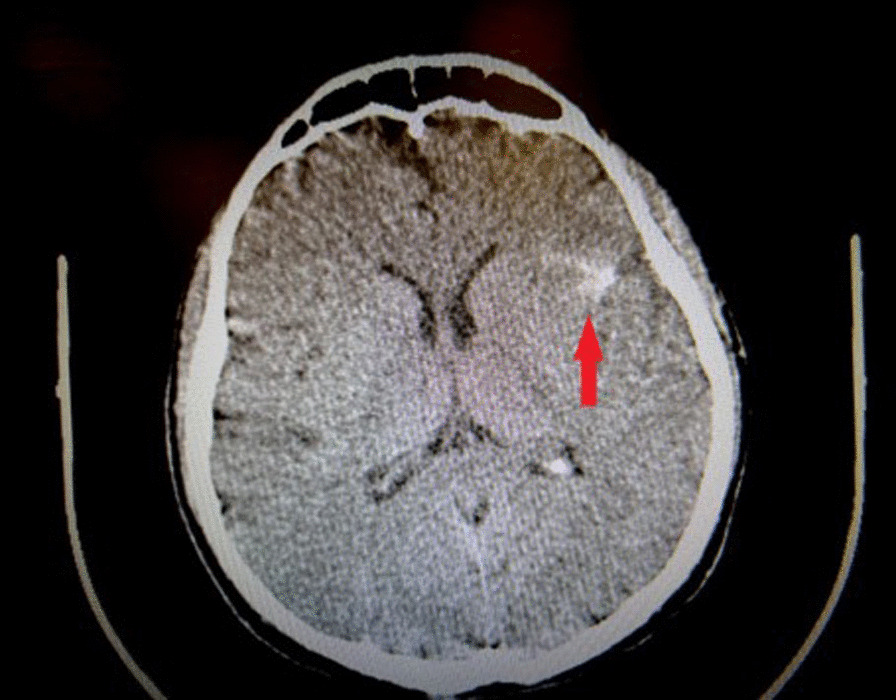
Axial cut of brain CT scan. Red arrow pointing to the SAH in left frontal and parietal lobes
